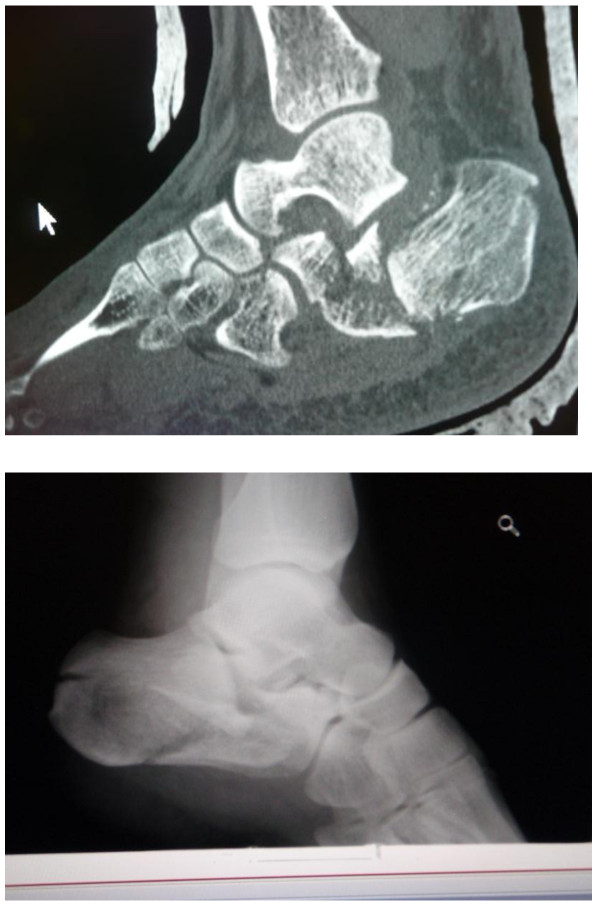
Case pair C, sagittal reconstruction of a CT-scan and a radiograph of the hind foot, showing complex fractures of the calcaneus of two passengers of the same armored personnel carrier after blast injury (improvised explosive device).
